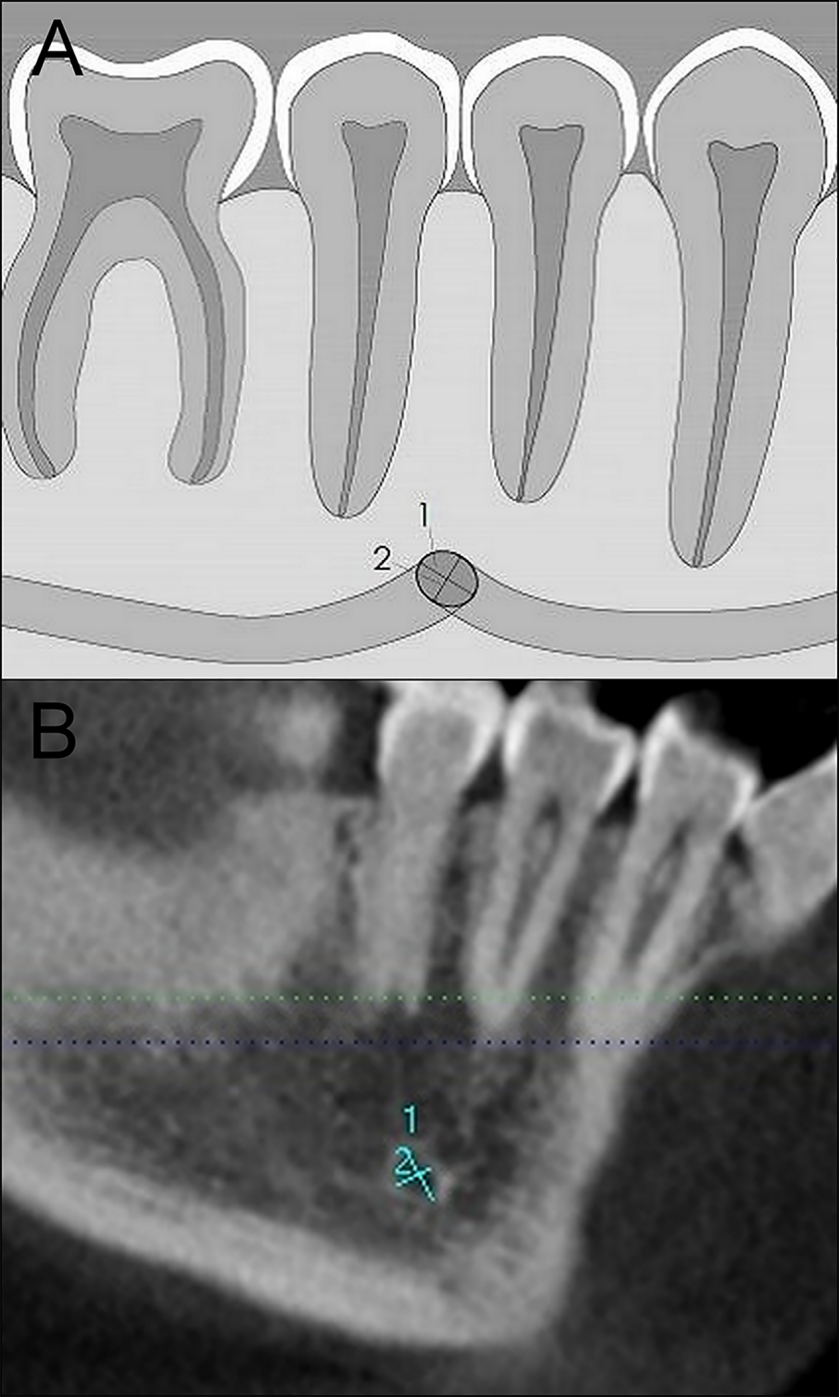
Dimensions of the MF. (A) Long diameter (1), Short diameter (2). (B) CBCT sagittal reconstructed slices of the mandible to show the long and short diameters of the MF.
